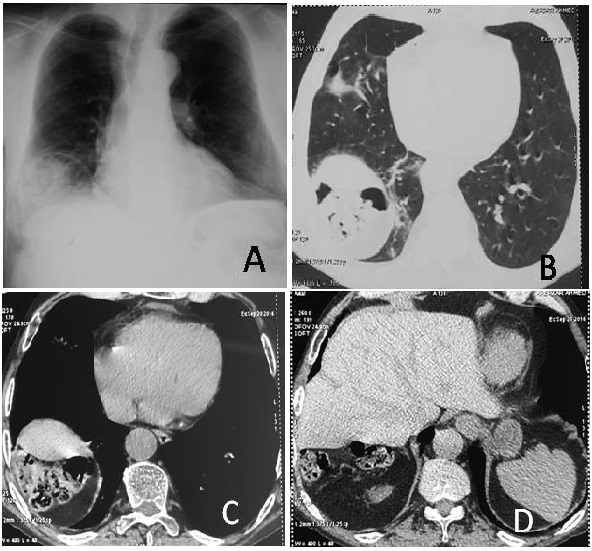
(A) posteroanterior chest films shows gas under elevated right hemidiaphragm; (B; C and D0; CT scan showing the interposition of right colic angle above the liver dome
